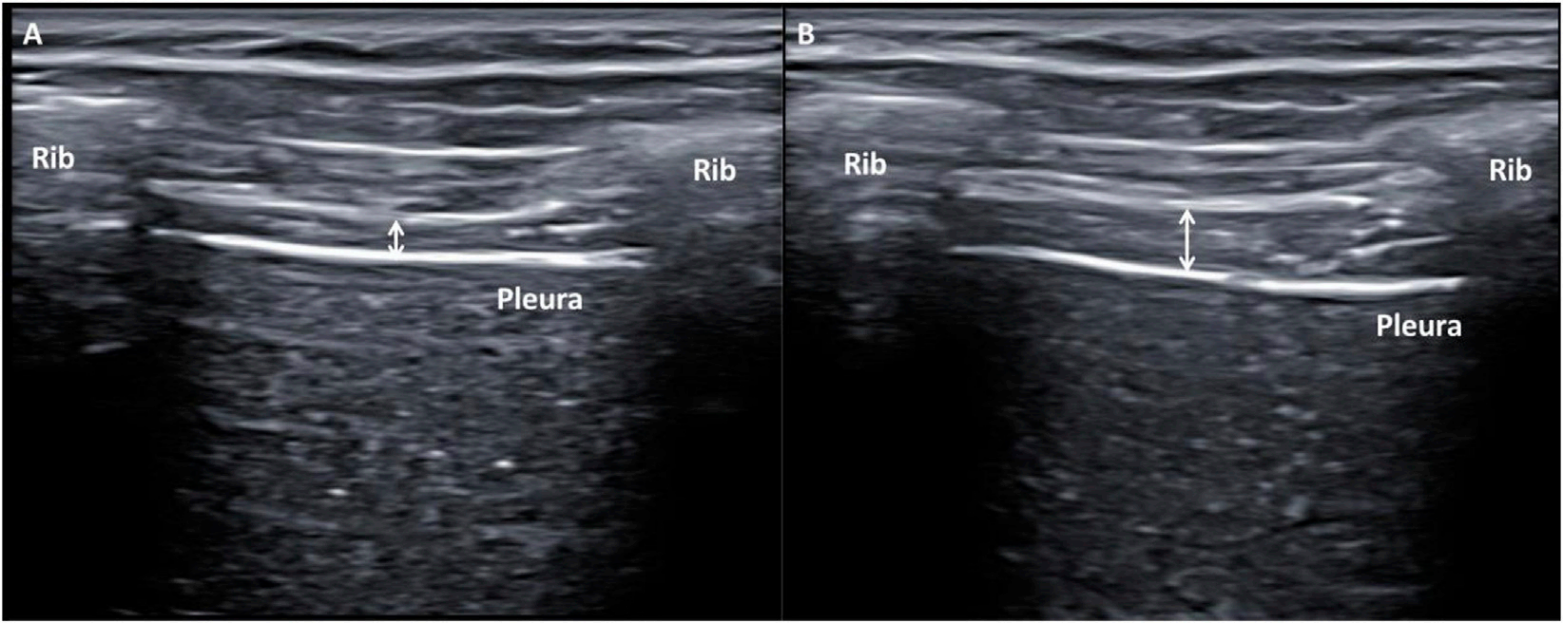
(B) Mode ultrasonography imaging of the hemidiaphragm thickness showing the last intercostal space following the mid-axillary line from the 12th rib upper edge to the 11th rib lower edge of the thorax region during normal breathing. (A) Hemidiaphragm thickness measurement marked by a white arrow during maximum expiration time (T^exp^). (B) Hemidiaphragm thickness measurement marked by a white arrow during maximum inspiration time (T^ins^).
